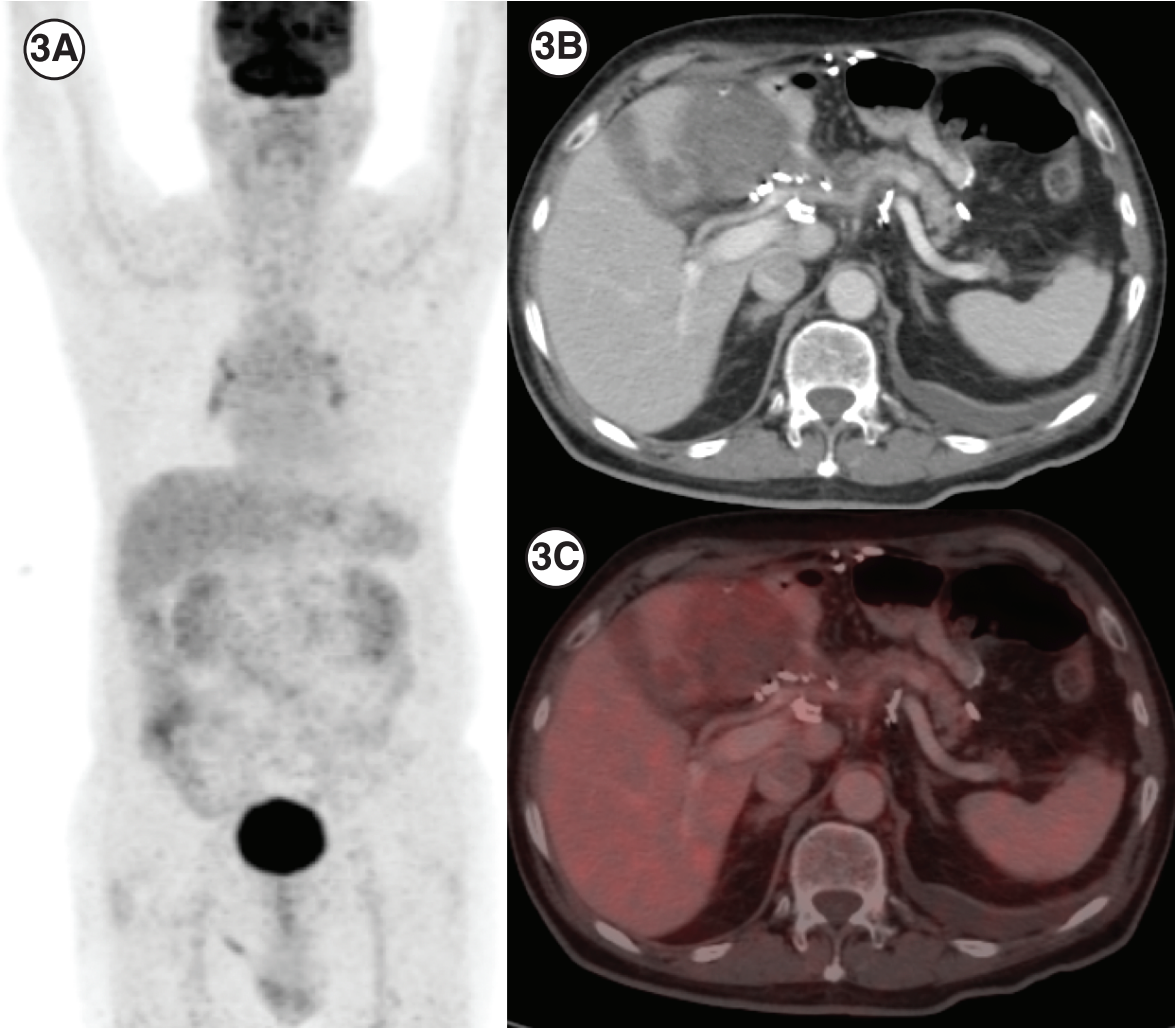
Response to avapritinib in case 5. (3A) In a patient with *PDGFRA* D842V mutation (patient E), maximum intensity projection image of ^18^F-Fluorodeoxyglucose (FDG) positron emission tomography/computed tomography (PET/CT) showing physiologic biodistribution in the brain, liver, kidneys and urinary bladder. (3B) Axial CT abdomen showing a heterogenous mass measuring 5.5 × 6.0 cm in the surgical bed in the gastro-hepatic region and a hypodense lesion in the segment IVB of the liver 2.1 × 2.0 cm with no significant FDG uptake in any of the lesions on fused PET-CT images, suggestive of partial response (3C).
